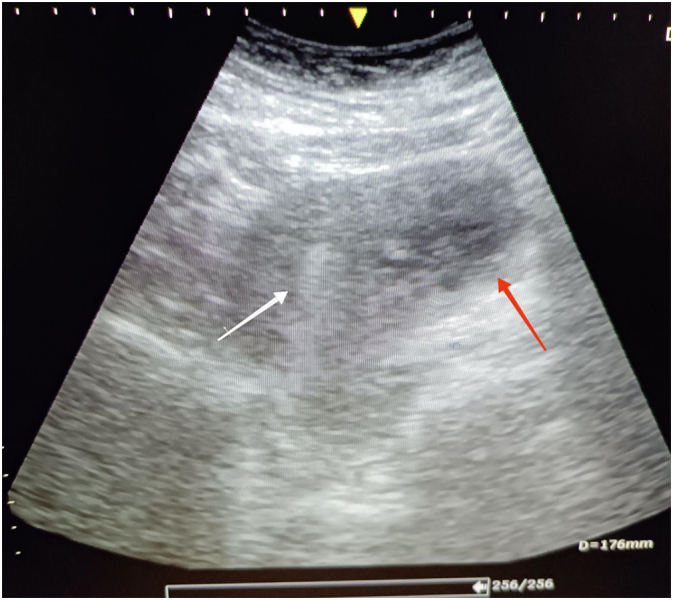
Transabdominal ultrasound showing the ectopic pregnancy occupying the right uterine horn separated from the endometrium (White arrow: endometrium, red arrow: gestational sac).
